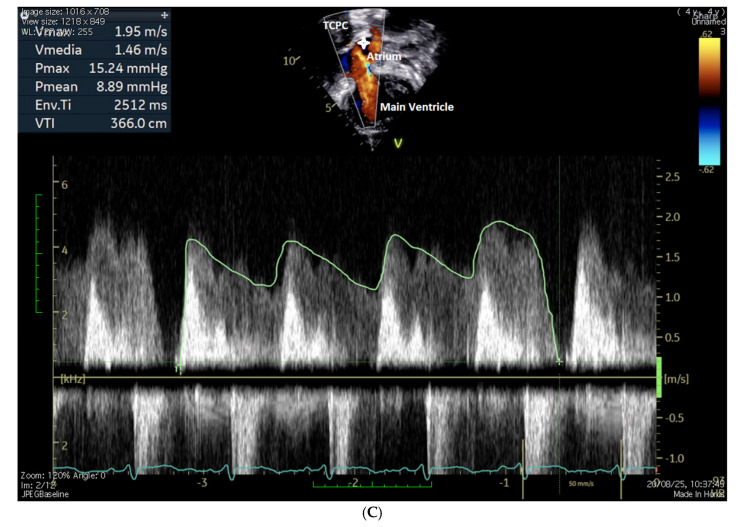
(A): Doppler of normal, phasic, and low-velocity (max velocity 0.4 m/s, mean velocity 0.2 m/s) TCPC conduct with respiratory variation; (B): Doppler of TCPC conduct with mild reversal; and (C): fenestrated TCPC with the gradient across the fenestration. TCPC = total cavo-pulmonary connection conduct; fenestration is arrowed.
